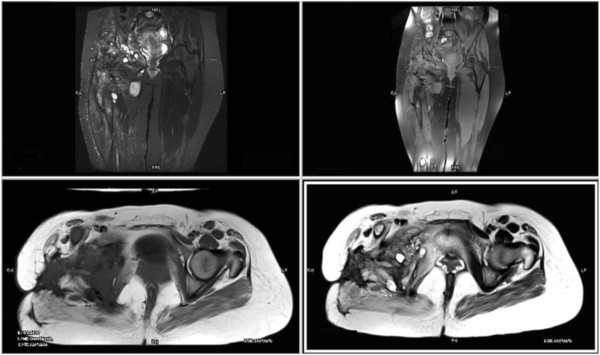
Coronal and axial magnetic resonance images of pelvis show heterogeneous signals on short-tau inversion recovery, predominantly high signals on T2-weighted image (fat saturation) and low signals on T1-weighted image.
